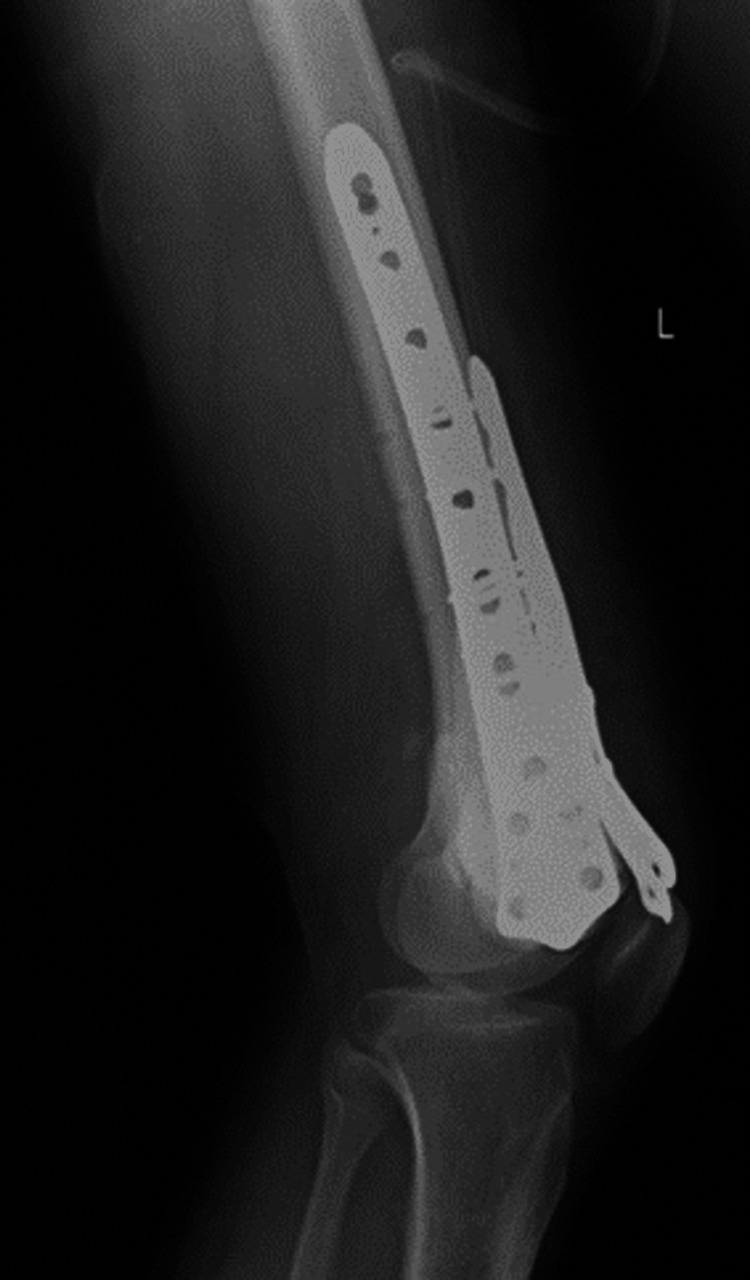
Postoperative plain radiograph, lateral view of the left knee with 3-plate fixation
